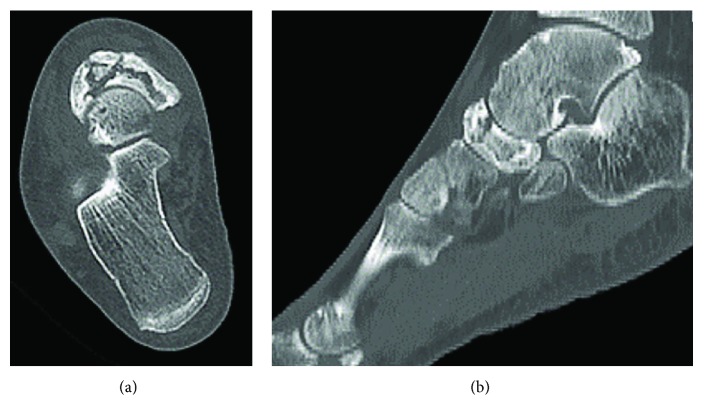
Axial and sagittal CT scans confirm fragmentation of the tarsal navicular and a cystic lesion in the talus.
